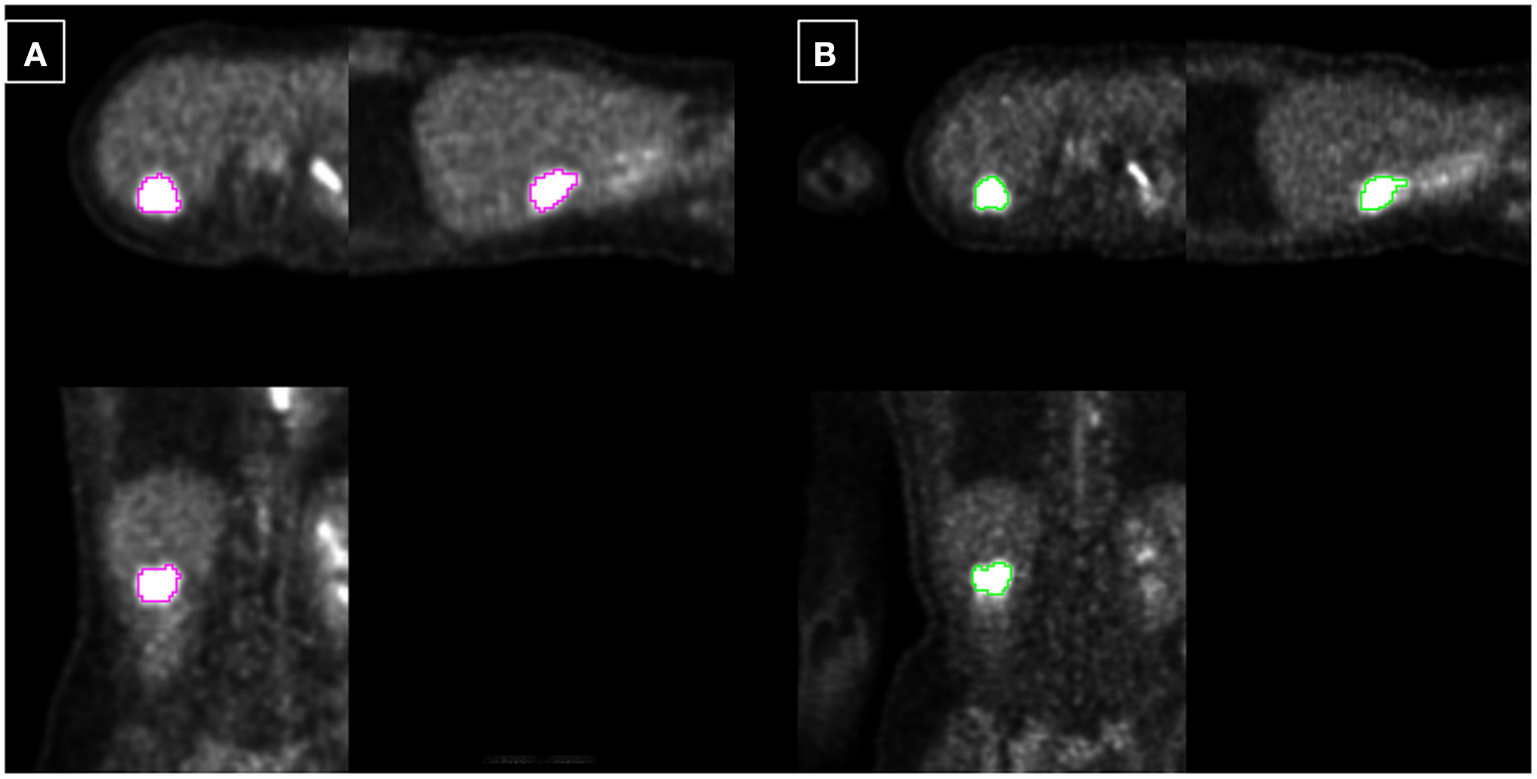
Example 1: 40-year-old female with liver metastasis of breast cancer. Each PET-CT (A) and PET-MR (B) was acquired 72 and 115 min after injection. The tumor is delineated based on fixed 40% threshold to SUVmax.
